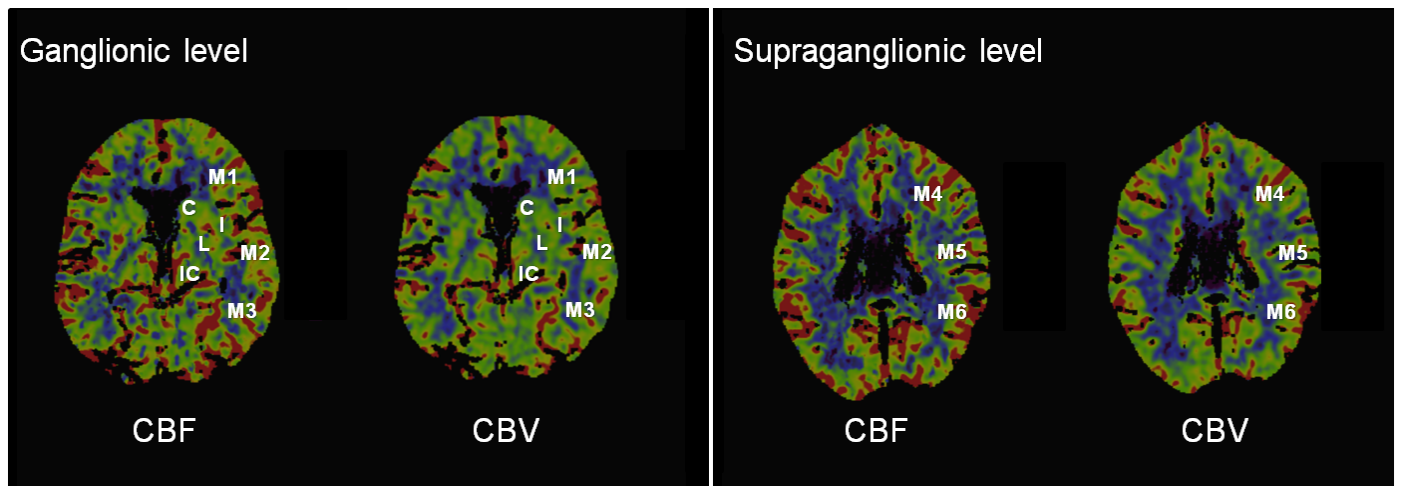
ASPECTS for mismatch assessment in CT perfusion. C, caudate head; I, insular ribbon; IC, internal capsule; L, lentiform nucleus; M1-M6, MCA region 1–6; CBF, cerebral blood flow; CBV, cerebral blood volume.
